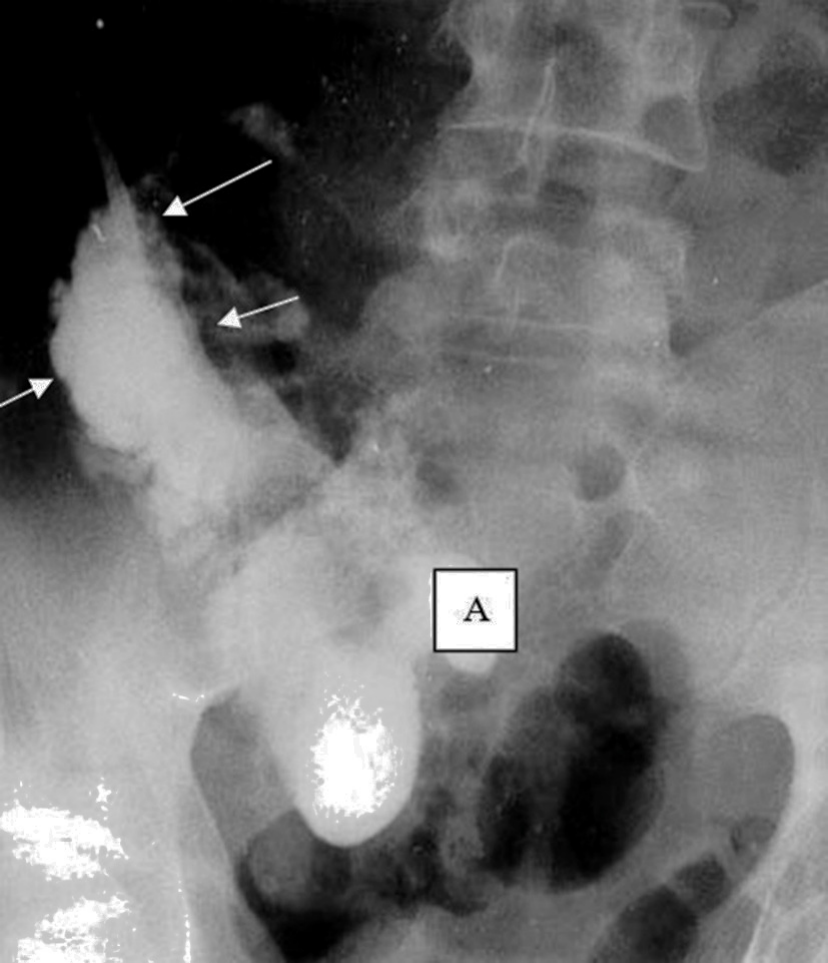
Ultrasound image of the abdominal abscess (arrows) and a sequestrum (A).
